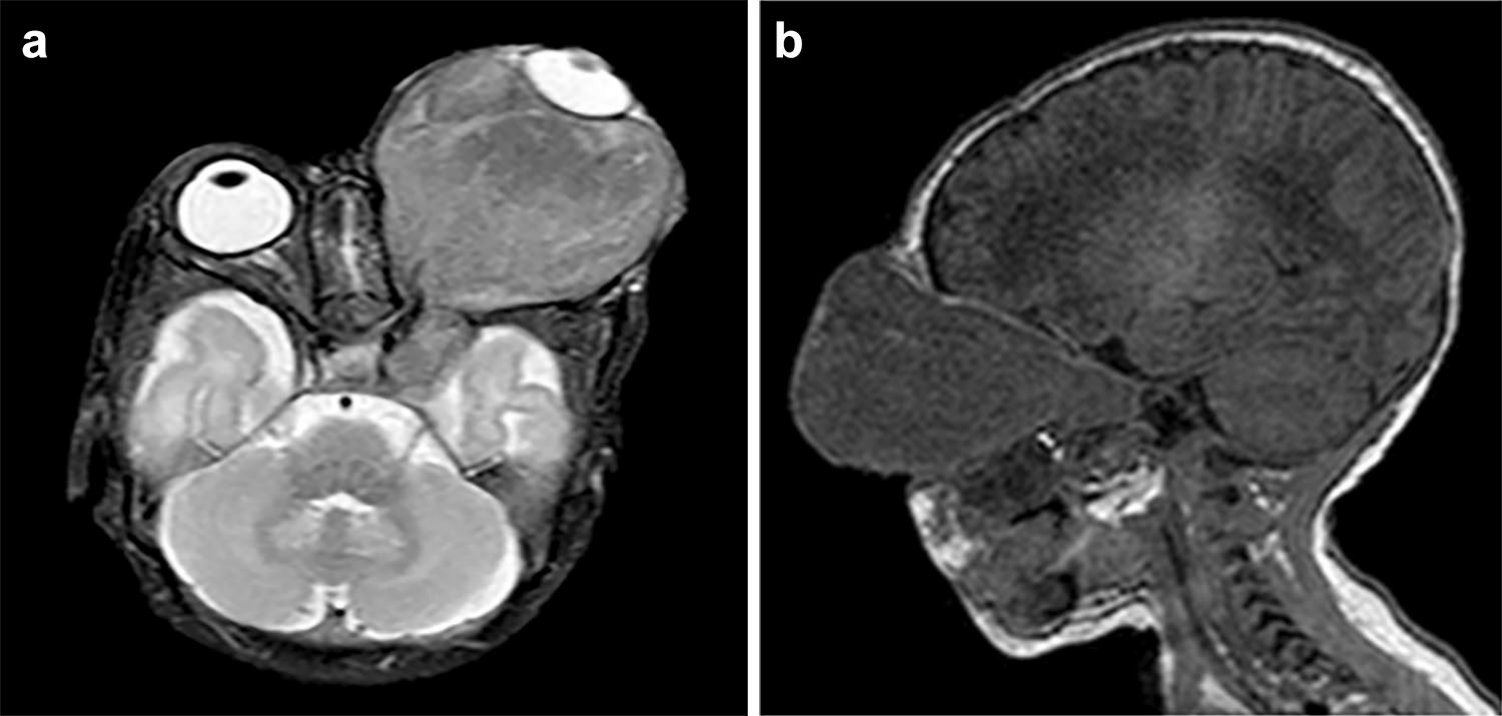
a MRI of the head demonstrated a large, well-circumscribed mass in the left orbit. b The mass measured 45 mm (anteroposterior) by 47.7 mm (transverse) by 50.9 mm (craniocaudal), with a 25-mm intracranial extension through a widened left optic canal
